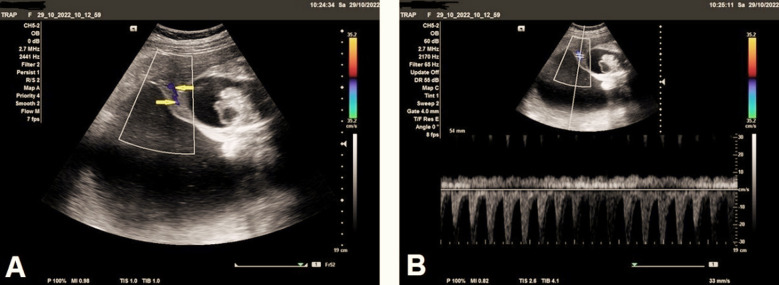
(A) Color Doppler examination shows acardiac twin with short umbilical cord containing only two-vessels (arrows), indicating single umbilical artery, and (B) Ultrasound Doppler examination shows acardiac twin with short umbilical cord containing only two-vessels, indicating single umbilical artery with reverse blood flow.
